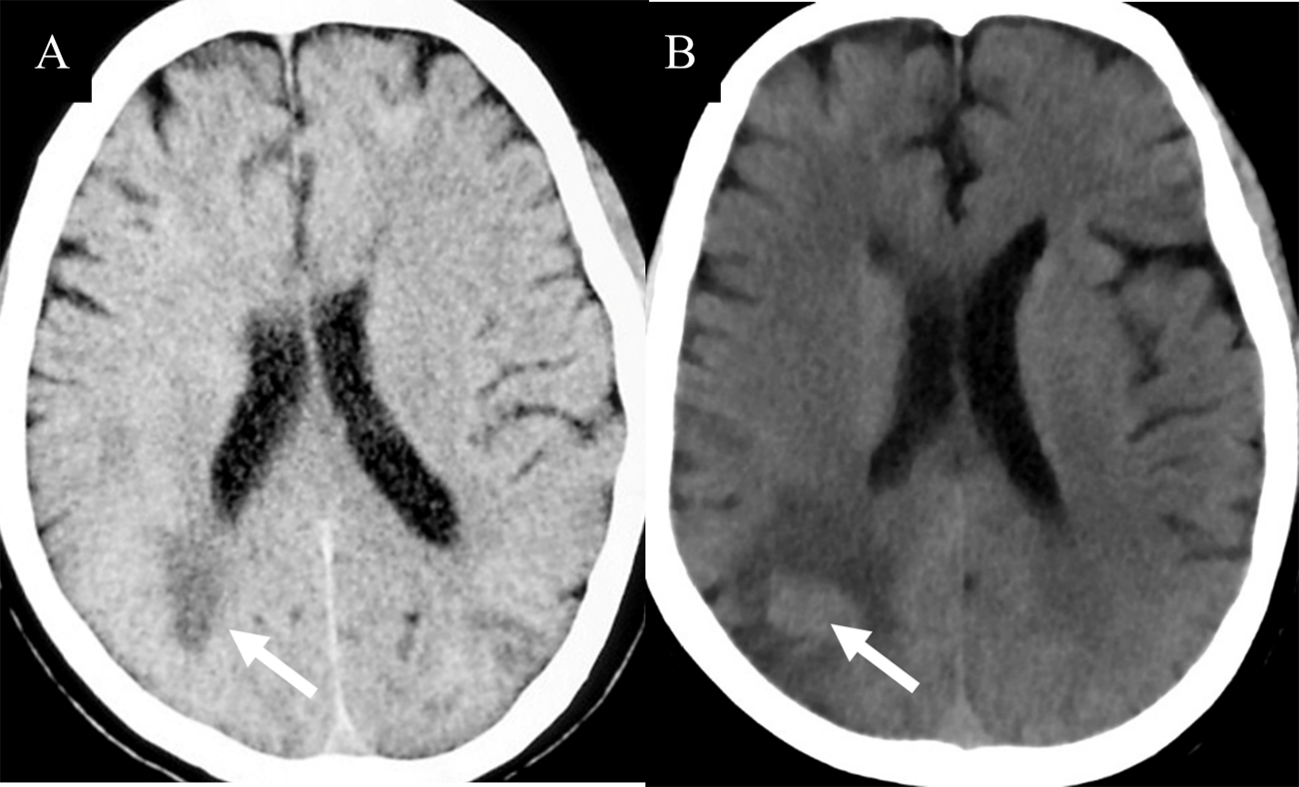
(A) Isodense lesion (white arrow) were detected on CT during an early disease. (B) One months later, brain lesions appeared hyperintense (white arrow). Pathological examinations indicated a diagnosis of PCNSL.
